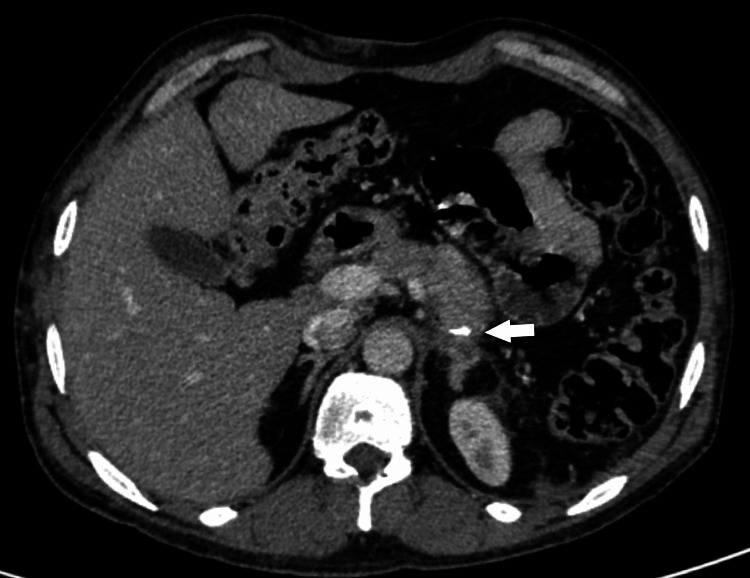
Axial CT showing post-operative changes with visible surgical staples
